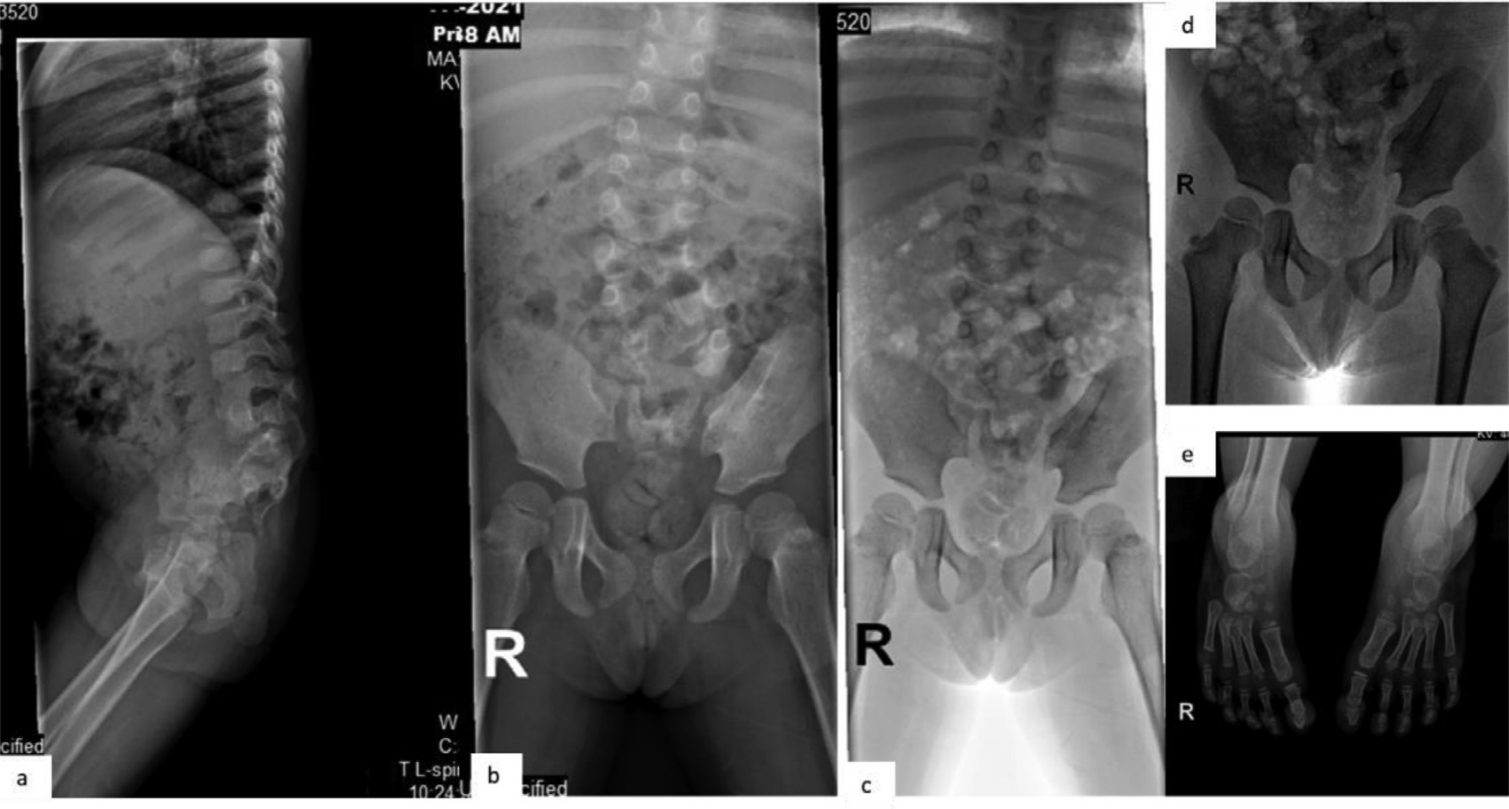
(A and B) Serial radiographs of the spine and pelvic bones display vertebral deformity with hemivertebrae in the lumbar segments and partial agenesis of the sacral bones, consistent with Pang Type IV as seen more clearly in the inverse technique (C and D). The iliac wings and pubic bones are present. (E) Bilateral foot deformity due to CTEV is also visible.
